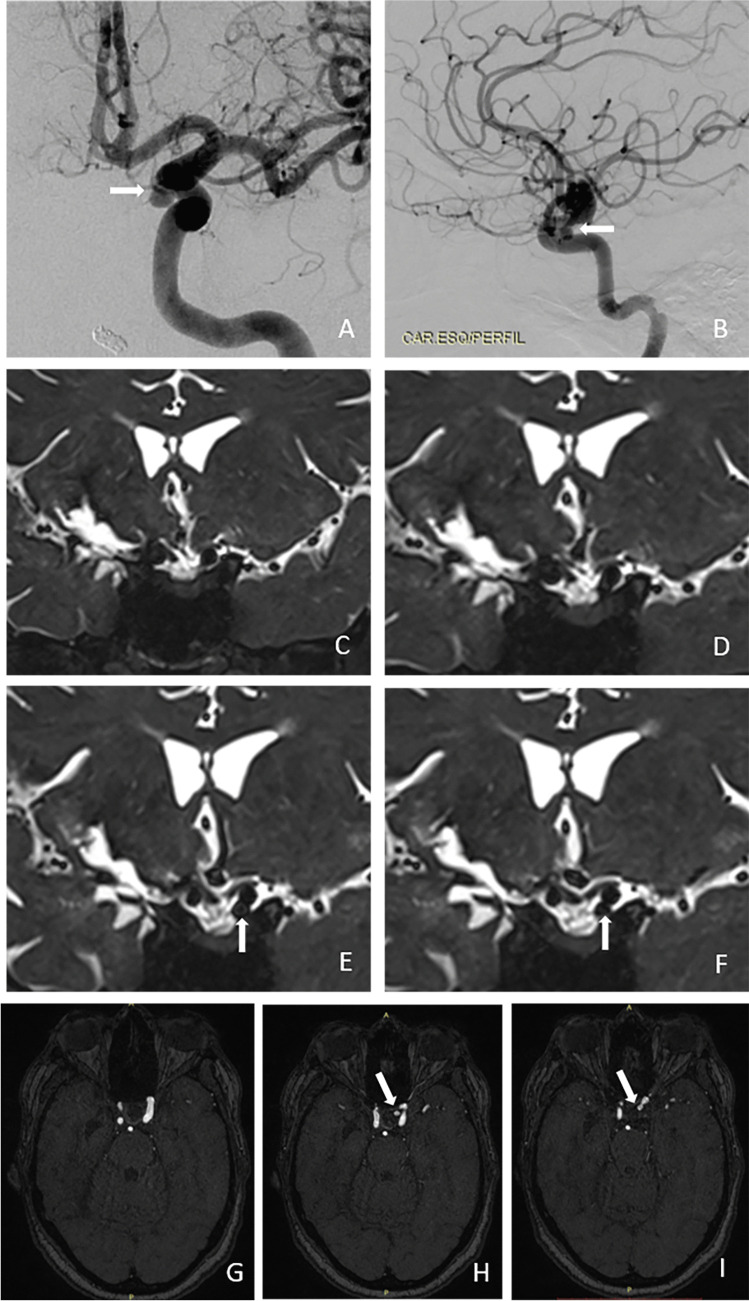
Cerebral digital subtraction angiography (DSA) of intradural aneurysm #22 (see Table 1) showing anteroposterior (panel A) and profile (panel B) views. Note the medial and inferior projection of the aneurysm (white arrows). Coronal T2-weighted magnetic resonance imaging (MRI) scans with a 3D fast spin-echo sequence showing anterior to posterior views of the anatomoradiological markers of the paraclinoid region and intradural aneurysm #22 (panels C–F). Note the hyper-intense signal from the cerebrospinal fluid (white arrows). In panel G–I, three-dimensional time-of-flight (TOF) magnetic resonance angiography was employed to enhance the view of the aneurysm (white arrows)
